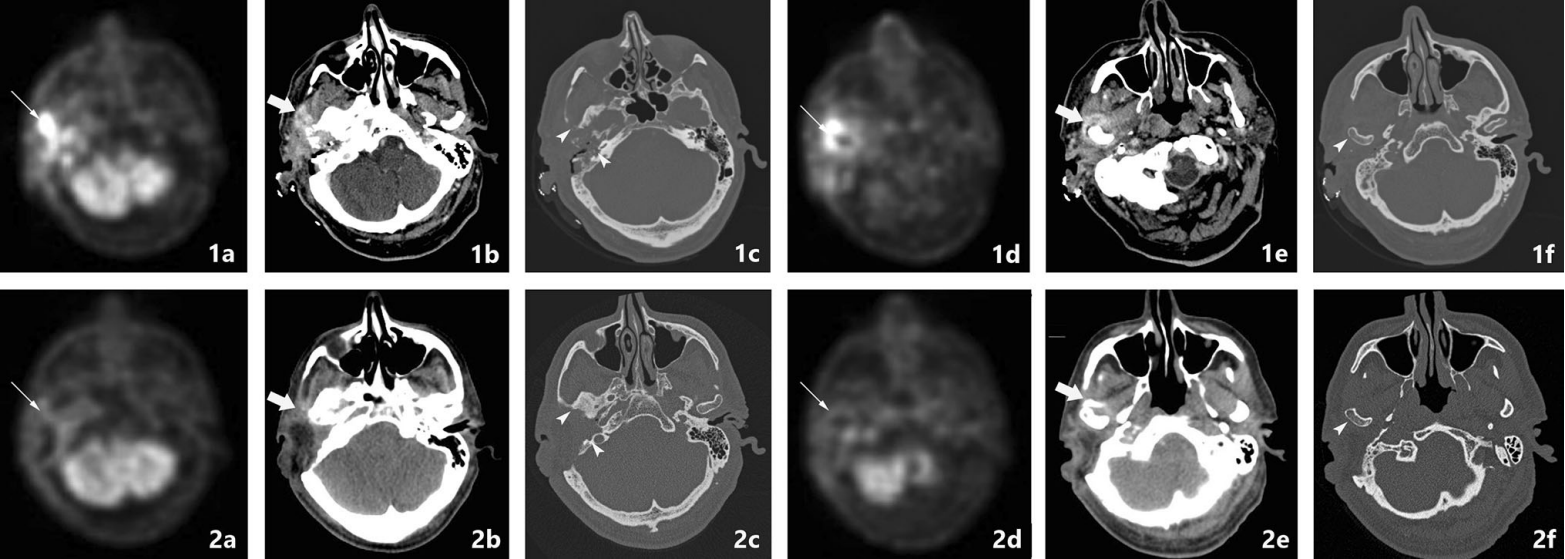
PET-CT follow-up. A 63-year-old male presented pain and otorrhea of the right ear for 3 months. Clinical symptoms included hearing loss and signs of vertigo. He was diagnosed with NOE. Initial FDG-PET-CT scan shows increased FDG uptake in the soft tissue in the subtemporal region expanding to the masticator space (thin arrow in image 1a) and the temporomandibular joint (thin arrow in image 1d). Corresponding CT in soft tissue setting diminishing of the fatplanes of the retromandibular space and masticator space, with enhancement of the peri-mandibular region and parotid region, corresponding with infectious spread (image 1b, 1e thick arrows). CT in bone window shows bone erosion of the temporal bone and mandibular condyle (image 1c,1f arrowheads). The patient received antibiotic therapy and a lateral temporal bone resection. Follow-up FDG-PET-CT scan shows markedly decreased FDG avidity (image 2a, 2d thin arrows), with decreased enhancement of soft tissue (image 2b, 2e thick arrows) as well as sclerotic margins of involved bone segments (image 2c, 2f arrowheads)
